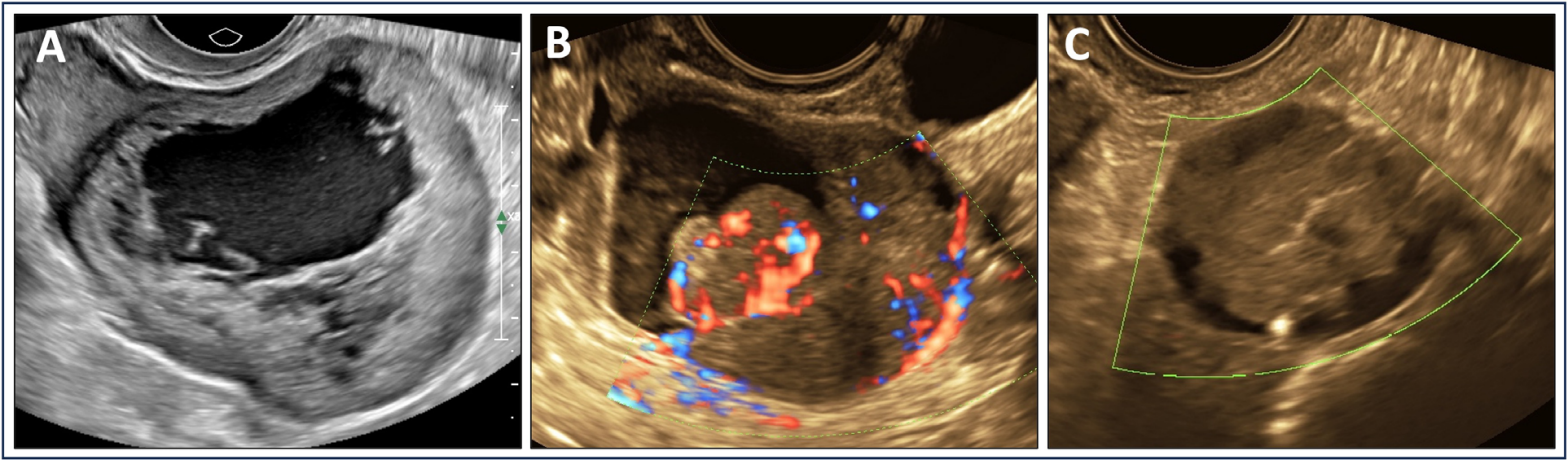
Rare borderline ovarian tumors. Clear cell and endometrioid BOTs are predominantly large solid tumors with small to large cystic areas on macroscopic examinations. Indeed, both the clear cell (A) and endometrioid (B) BOT cases showed large solid components with cystic areas and high vascular flow on Doppler imaging. Brenner BOTs macroscopic appearance is often described as cystic lesions with papillary projections. In this case (C), a cystic lesion with what appears to be a large papillary projection is present.
